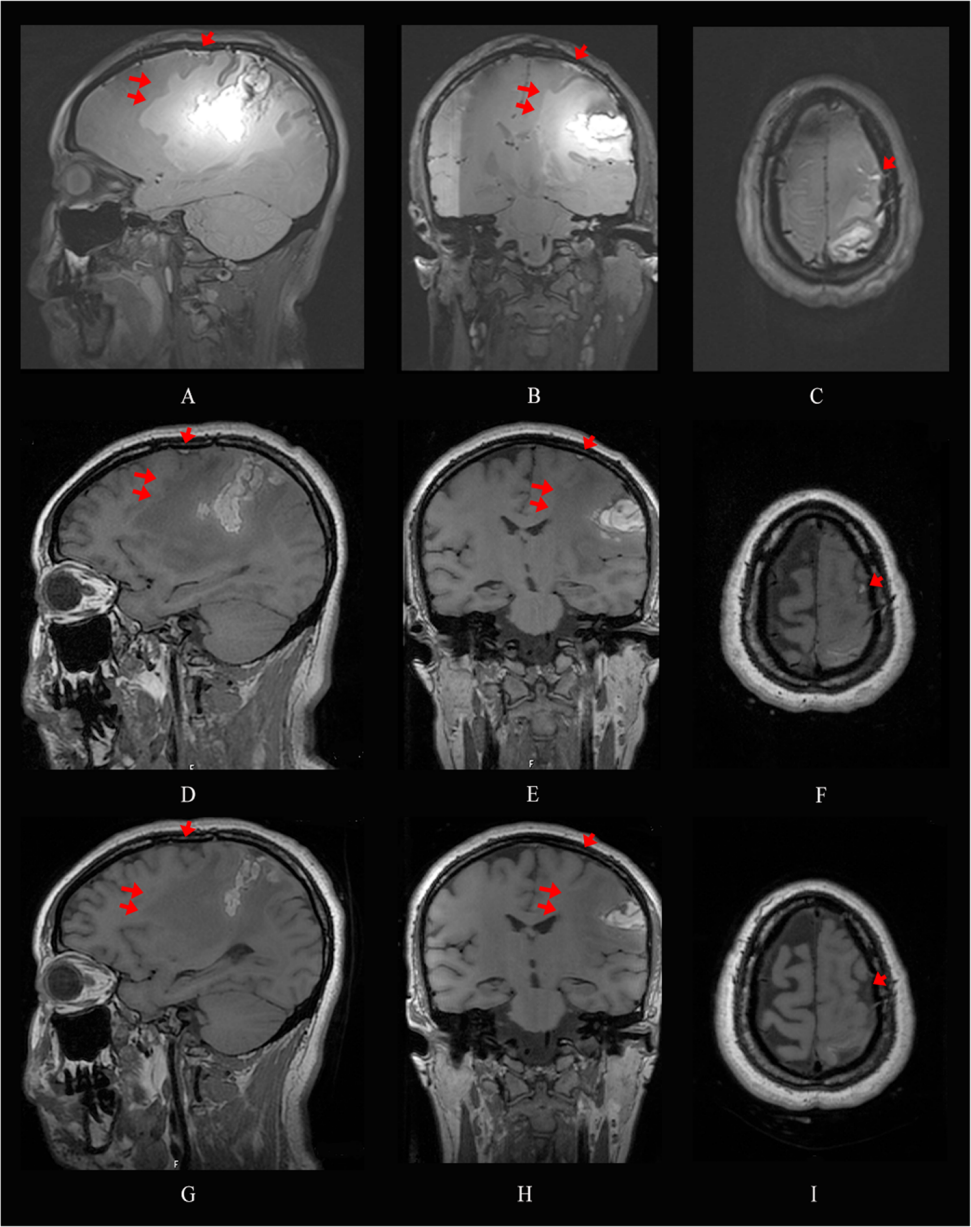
Head MRI ten days after admission showed isolated cortical vein thrombosis (arrow head) over the frontal cortical surface and expanding hematoma and edema (double arrow head) that caused cingulate herniation in the sagittal, coronal and axial section (a-c) on T2 SPACE sequence. The sizes of thrombosis (arrow head), hematoma and edema (double arrow head) continuously decreased on T1 CUBE sequence after five (d-f) and twelve (g-i) days of anticoagulation, respectively
